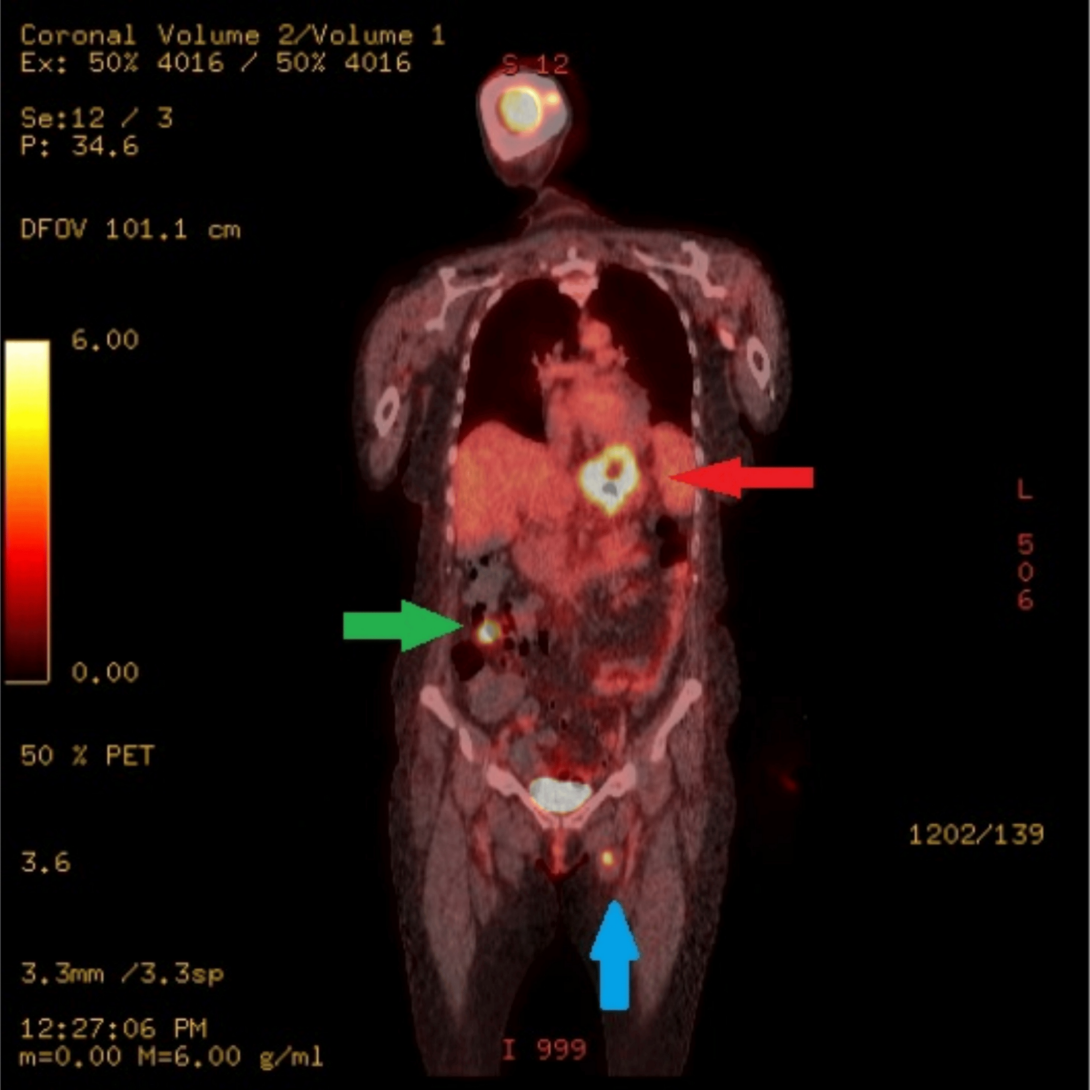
PET/CT. FDG avid necrotic distal esophagus and gastric fundus lesion (red arrow), SUV max 14, consistent with biopsy-proven adenocarcinoma of the esophagus. Focal FDG avidity along the proximal left adductor muscle (blue arrow), SUV max 6.49, representing intramuscular metastasis. FDG avid left adrenal nodule measuring up to 2 x 1.5 cm, SUV max 7.02, likely metastatic in etiology. FDG avid right lower quadrant peritoneal soft-tissue attenuation lesion measuring 2.4 x 1.8 cm, SUV max 8.7, likely reflective of peritoneal metastasis (green arrow) PET/CT: positron emission tomography/computed tomography; FDG: fludeoxyglucose F18; SUV max: maximum standardized uptake value
